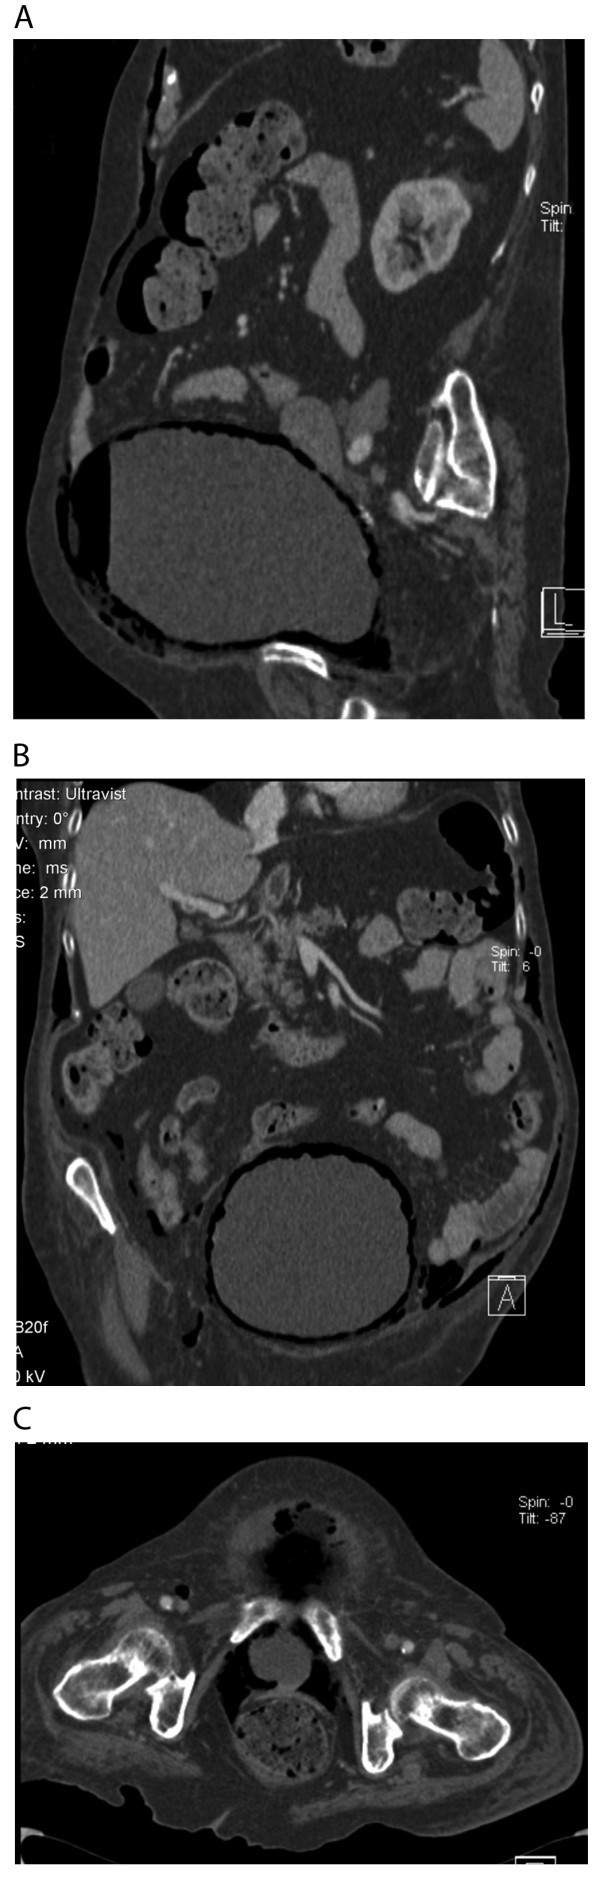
Radiographic imaging of subcutaneous emphysema secondary to emphysematous cystitis. a CT sagittal section showing gas within the anterior abdominal wall and within the bladder lumen and wall. b CT coronal section showing gas within abdominal wall outside the muscle layer, intra-abdominal but retroperitoneal, and within the bladder wall. c CT axial section showing gas within the bladder base and femoral canal.
